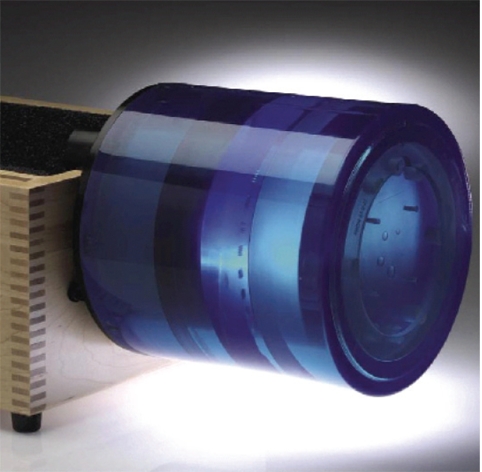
Catphan-504 is designed to evaluate the performance of axial and spiral CT scanners with enhanced sensitometry samples for radiation therapy planning. Its modular design includes test modules CTP404 (slice geometry & contrast linearity), CTP528 (high resolution), CTP515 (low contrast), and CTP486 (uniformity) modules.
